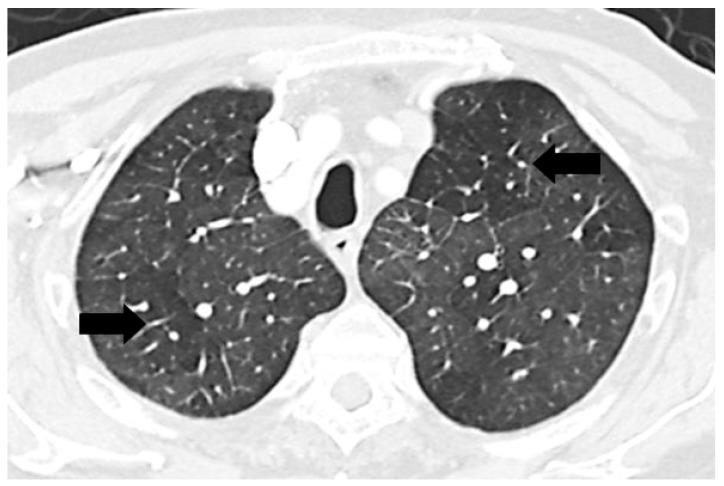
Chest computed tomographic (CT) scan demonstrates mosaic pattern of lung attenuation where there is a patchwork of regions of differing attenuation with well-defined borders (black arrows).
